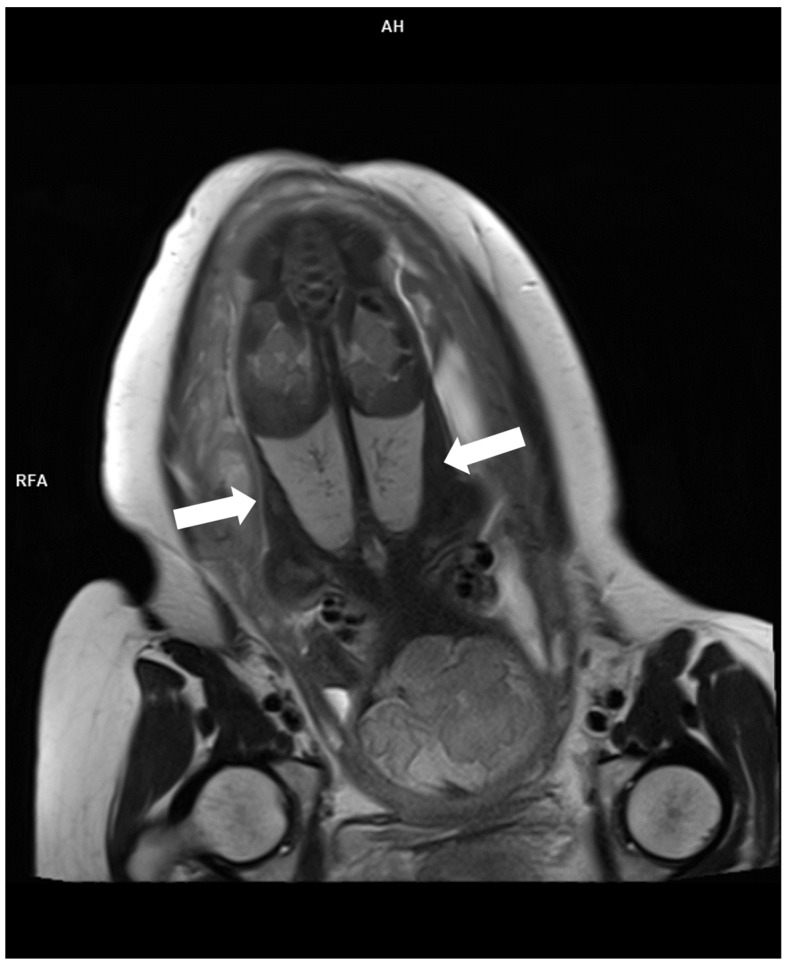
T2W HASTE image of fetal MRI at 32nd week of gestation clearly depicting the lungs (white arrows), enabling segmentation and accurate estimation of lung volume, helpful in predicting lethality of skeletal dysplasia.
